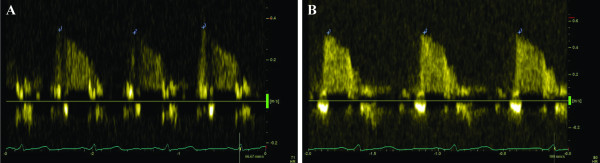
An example of impaired CFR. Coronary flow by spectral Doppler at rest (A) and during hyperaemia (B). CFR < 1.5, estimated in the patient with absence of myocardial viability and with extensive myocardial perfusion defect.
